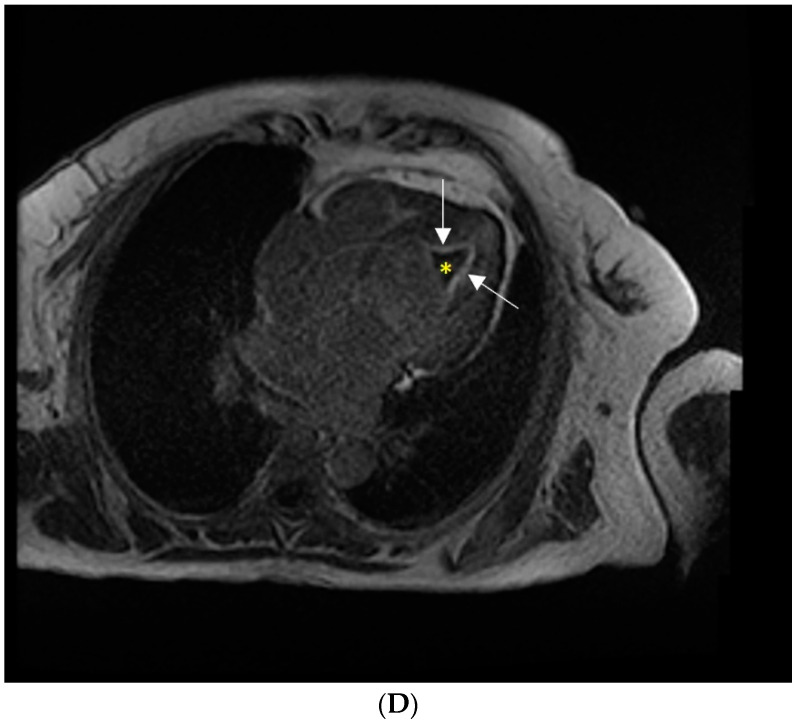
(A) Echo Parasternal long axis view showing obliteration of the left ventricular apex. (B) Echo Parasternal long axis view: normal-size left ventricle with normal systolic function, increased thickness of the posterior mitral leaflet and chordae, apical obliteration. (C) Cardiac MRI: 4 chamber view showing a normal-size left ventricle, with preserved ejection fraction and an apical mass engulfing the papillary muscles. (D) Cardiac MRI: inversion recovery images 10 min after contrast injection showing left ventricular apical thrombus (white arrows) and sub-endocardial enhancement (star).
